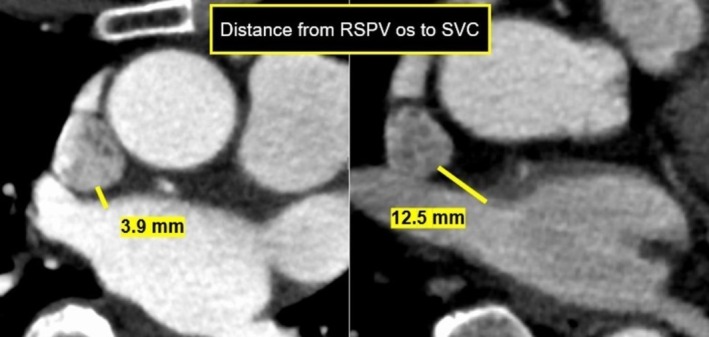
CT analysis revealed: Case 6: RSPV‐SVC shortest distance = 12.5 mm (right). Case 7: RSPV‐SVC shortest distance = 3.9 mm (left). The SVC height was defined as the level of the LA roof, and evaluated on axial CT images.
